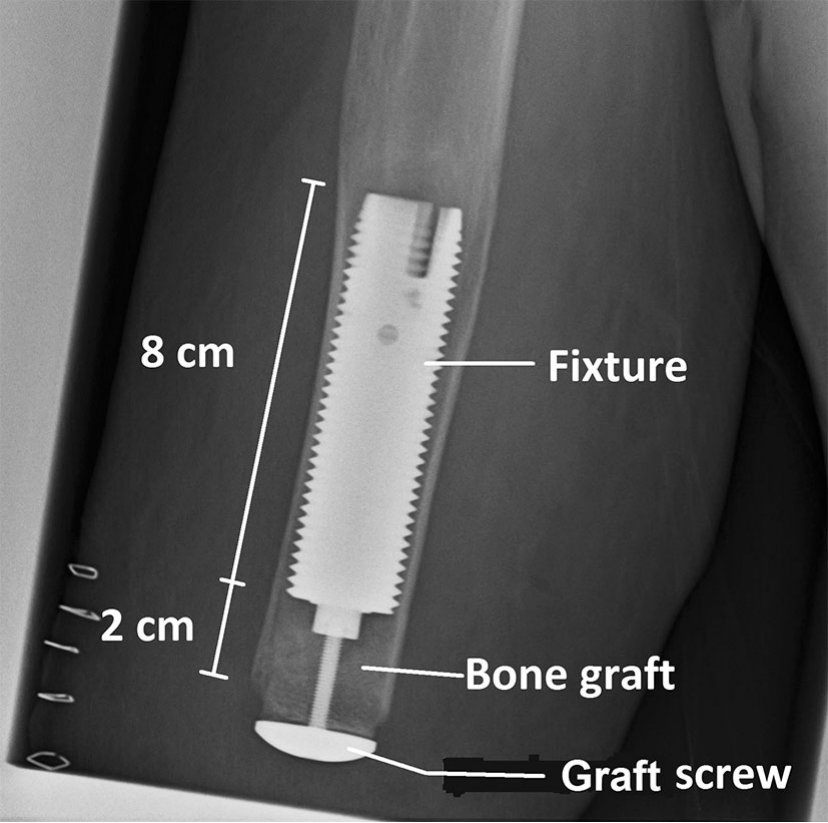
Direct postoperative radiograph is shown after S1. The fixture is countersunk by 2 cm into the medullary cavity and the residual space at its distal end is filled by autologous bone graft, which is held under compression by the so-called “graft screw.” At S2, the graft screw is removed and the healed bone graft is drilled to a diameter equal to the diameter of the abutment.
